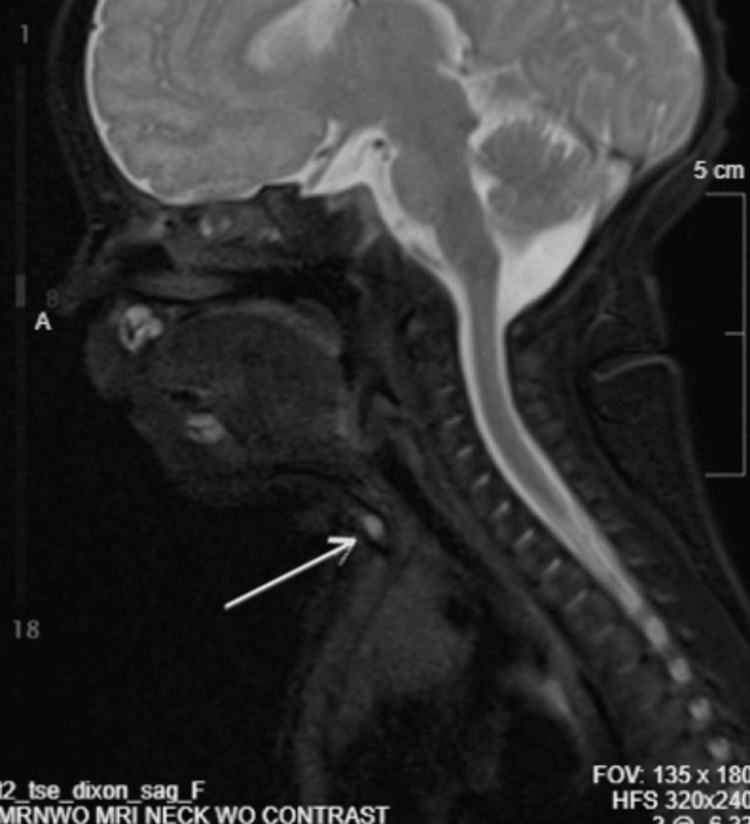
Sagittal MRI of the neck (alternate sequence) demonstrating a superficial infrahyoid midline lesion (arrow) without deep extension, consistent with congenital midline cervical cleft. MRI: magnetic resonance imaging
